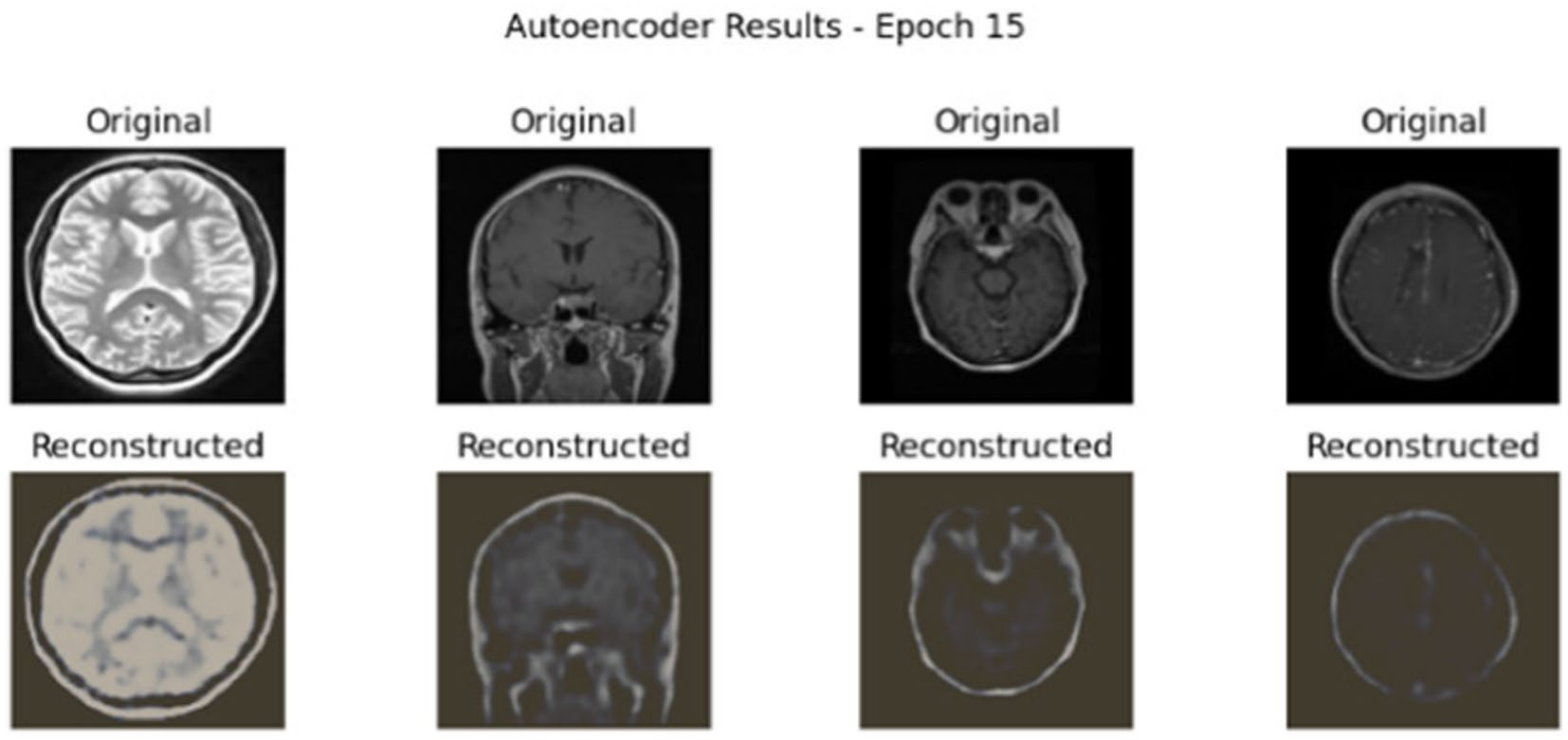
AE reconstruction results for MRI brain scans at epoch 15 on Dataset I.
